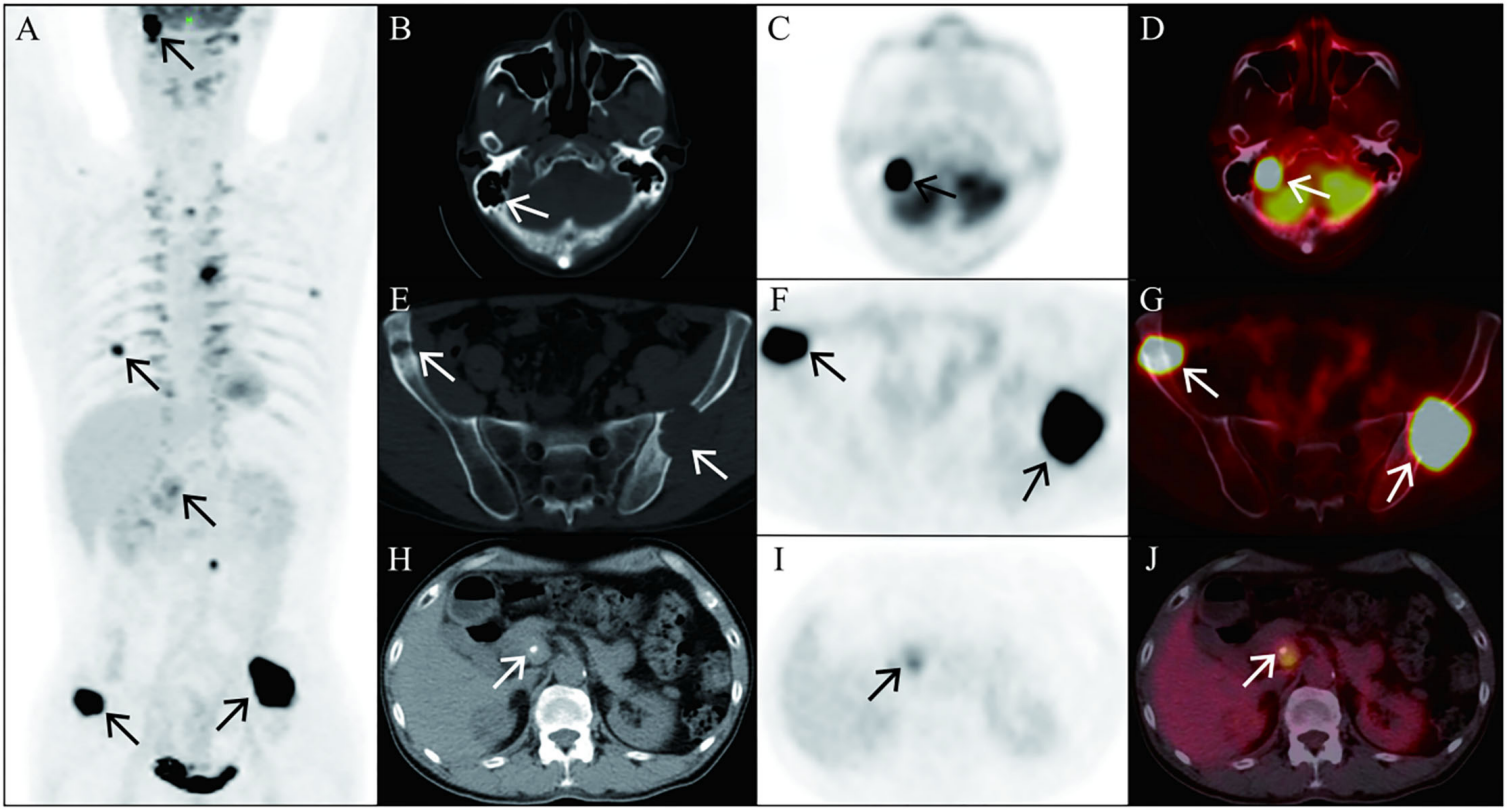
As shown in MIP of 18F-FDG PET, 18F-FDG uptake in the petrous part of right temporal bone [(A), arrows], right foramen magnum, right four anterior ribs, left five lateral ribs and posterior ribs, left eight posterior ribs [(A), arrows], left pedicle of Lumbar 3 vertebral body [(A), arrows], and bilateral ilium [(A), arrowhead] are revealed and characterized with osteolytic bone destruction. Axial CT, PET, and fusion PET/CT images of representative involve bone lesions, including the petrous part of right temporal bone [(B–D), arrows; SUVmax, 20.3] and bilateral ilium [(E–G), arrowhead; SUVmax, 20.3], which are exhibited. In addition, the image shows the 18F-FDG uptake in the abdominal lymph nodes peri-pancreatic, indicating pheochromocytoma metastasis [(H–J), arrow; SUVmax, 5.2].
